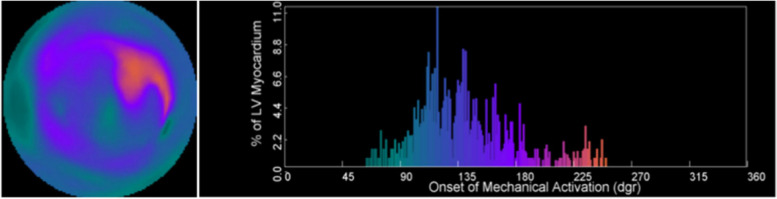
An example of a 64 years old male patient with AHF (LVEF = 46.8%) died after followed up 22 months. PBW (164°) was measured by the phase analysis of SPECT MPI. Reprinted with permission from ref. 24. Copyright (2021) Journal of Nuclear Cardiology
